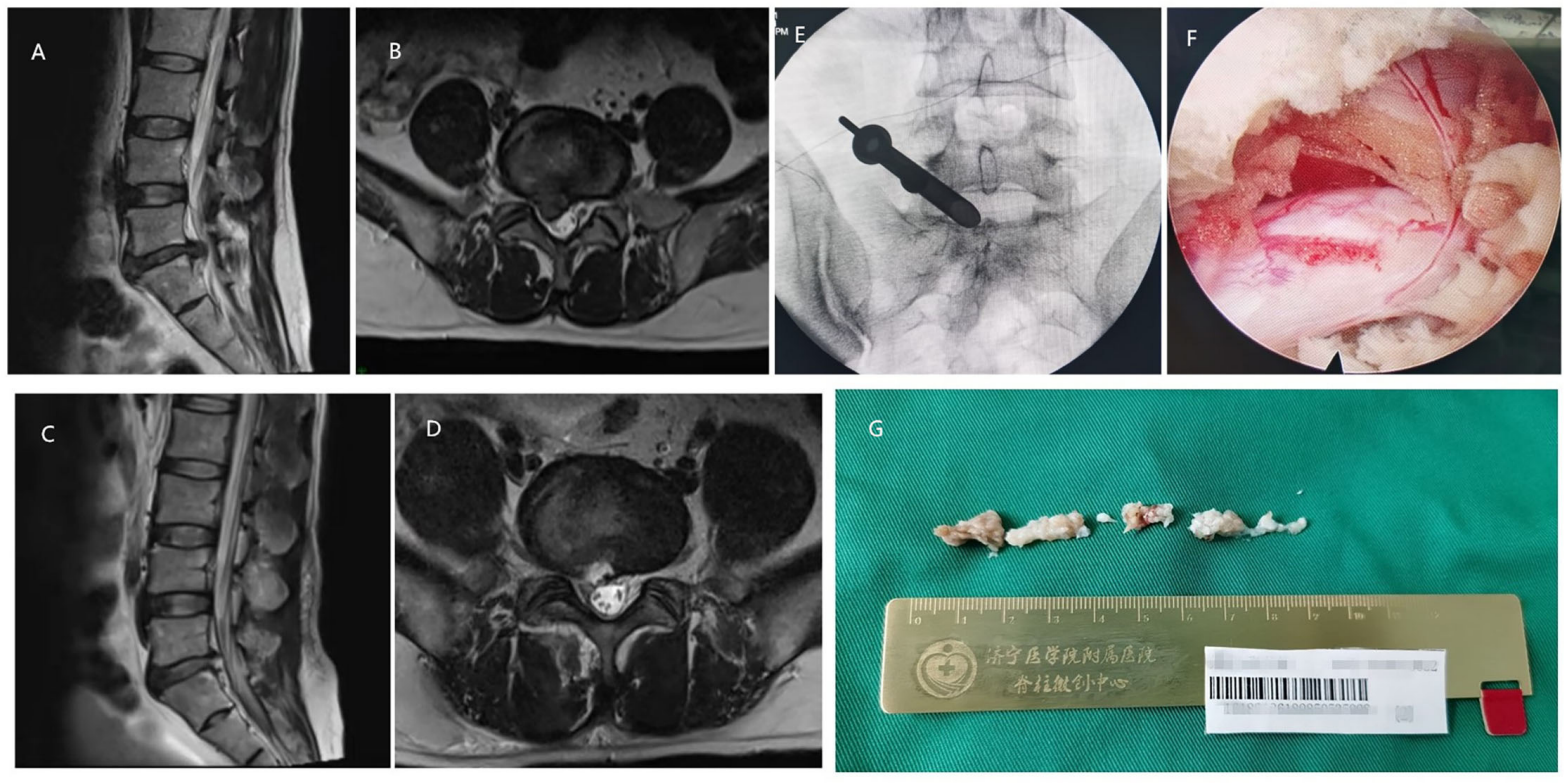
A 56-year-old male with the L5/S1 LDH underwent PELD with the approach of interlaminar. (A,B) Preoperative MRI showed LDH was located at the L5/S1 level. (C,D) Postoperative MRI revealed the herniated nucleus pulposus tissues were removed and the nerve root was decompressed. (E) Intraoperative C-arm fluoroscopy showed the location of the working channel. (F) The decompressed nerve root under endoscopic view. (G) Resected nucleus pulposus tissues in the operation.
